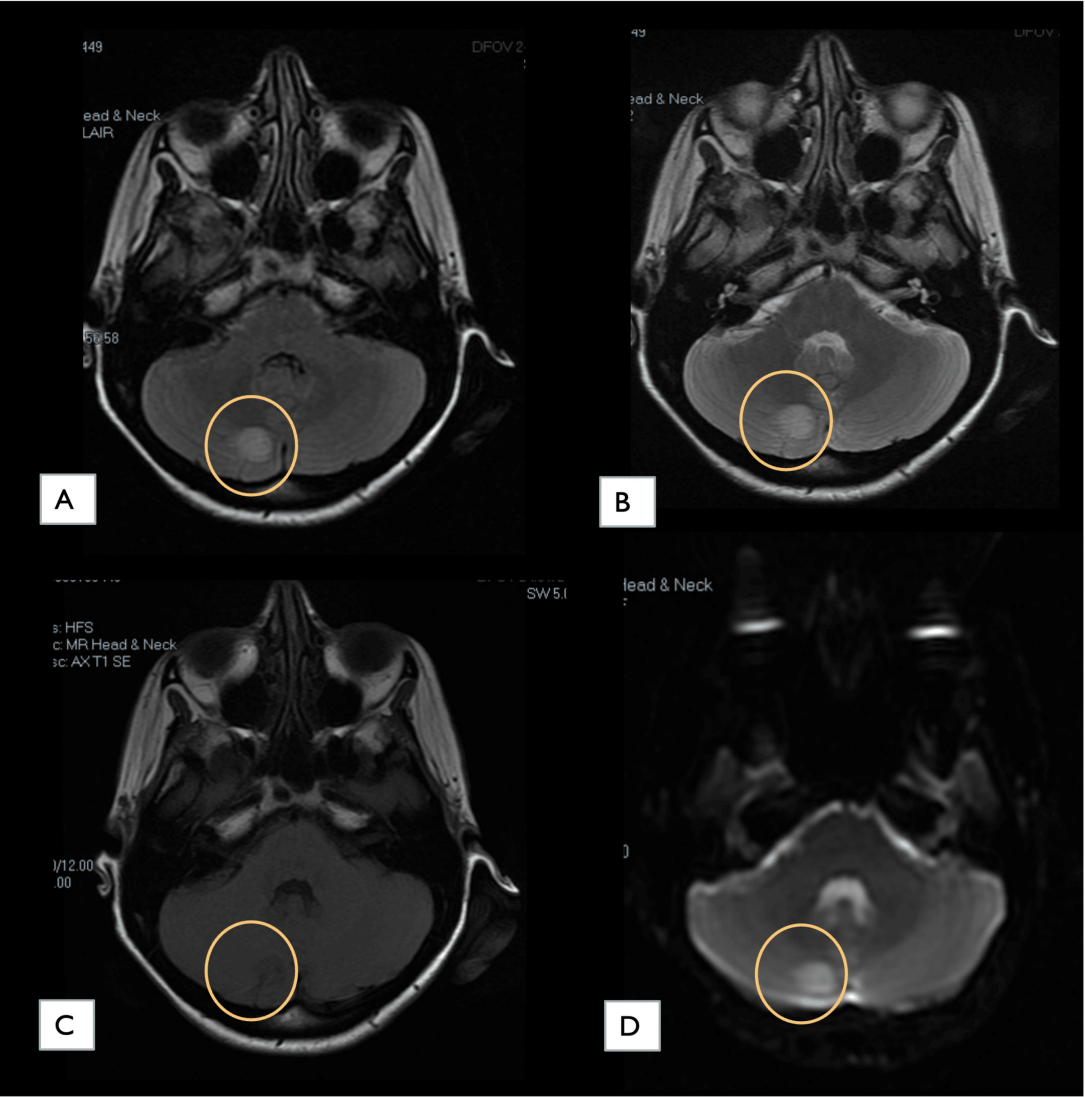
Brain MRI images showing an altered signal intensity along the posterior aspect of the right cerebellar hemisphere. In FLAIR (A) and T2-weighted (B) images, it appeared as a localized hyperintensity. In T1-weighted images, it appeared as subtle hypointensity (C). Mild signal restriction was observed in the DWI sequence (D). Considering the patient’s clinical results, encephalitis was determined to be the top primary diagnosis. FLAIR: fluid-attenuated inversion recovery; DWI: diffusion-weighted imaging
